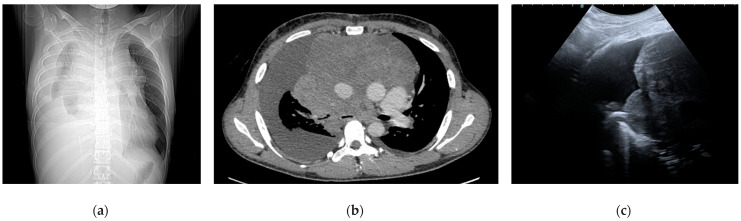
Primal mediastinal Hodgkin lymphoma. (a) Scout image demonstrating a right pleural effusion associated with mediastinal enlargement. (b) A contrast-enhanced tomographic image shows a large prevascular mediastinal mass with airway compression, heart dislodgement, and right pleural effusion. (c) Ultrasound appearance of the mass and the pleural effusion from a right parasternal view. The images are owned by the Department of Pulmonology, S. Maria della Misericordia University Hospital, Udine, Italy. Informed consent was obtained from the patients for the publication of the images in an anonymized format.
